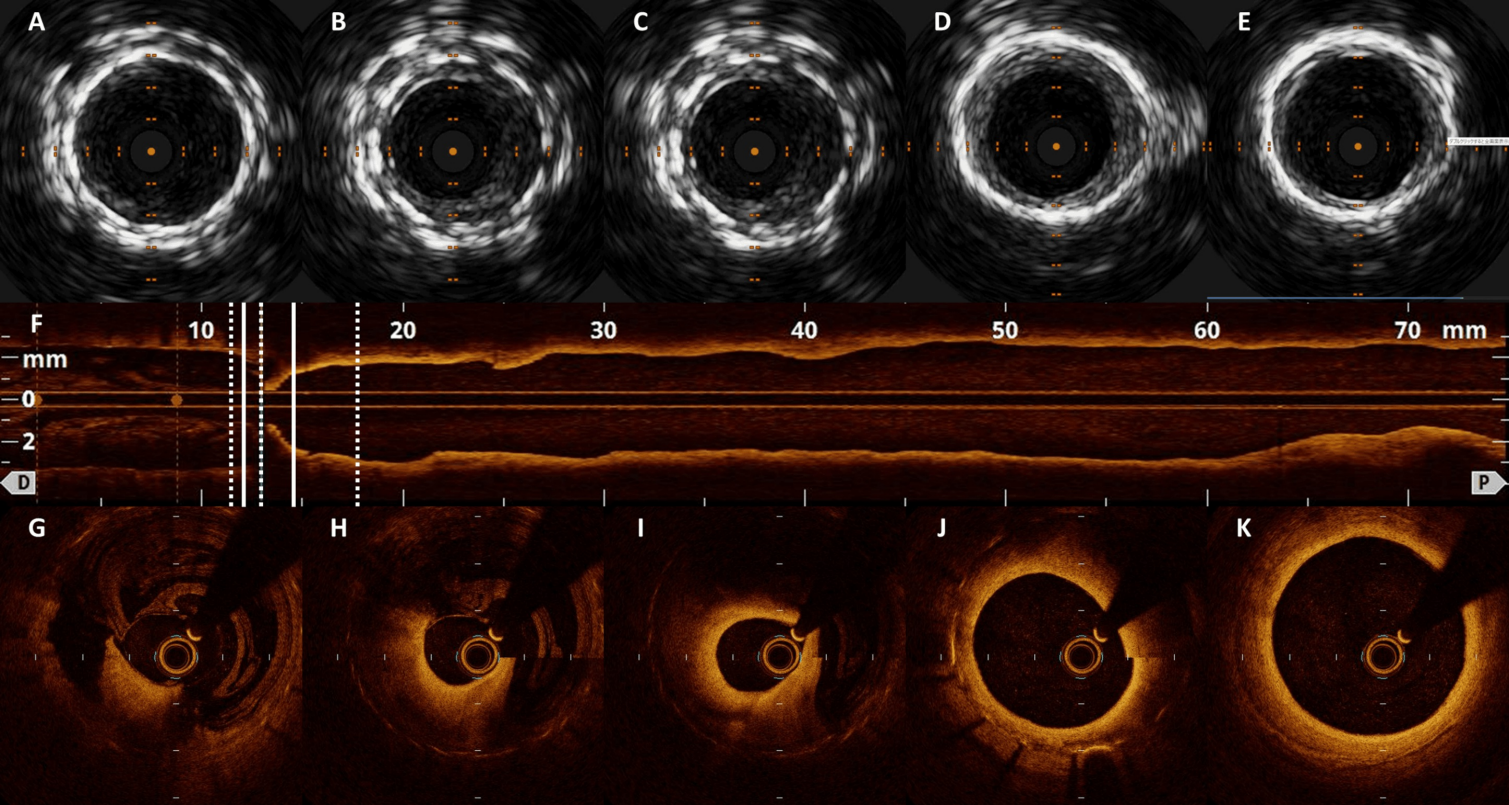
The images of IVUS and OCT. The images show observations from distal to proximal in the order of A-E, G-K. Corresponding positions in OCT image F are shown for A and G, B and H (A-E) IVUS identified thin, low-signal neointimal hyperplasia with luminal area of 9.1 mm^2^. (F-K) OCT showed neointimal proliferation, resulting in significant stenosis with a luminal area of 1.59 mm^2^. Approximately 180° was not in contact with the vessel wall. IVUS, intravascular ultrasound; OCT, optical coherence tomography
